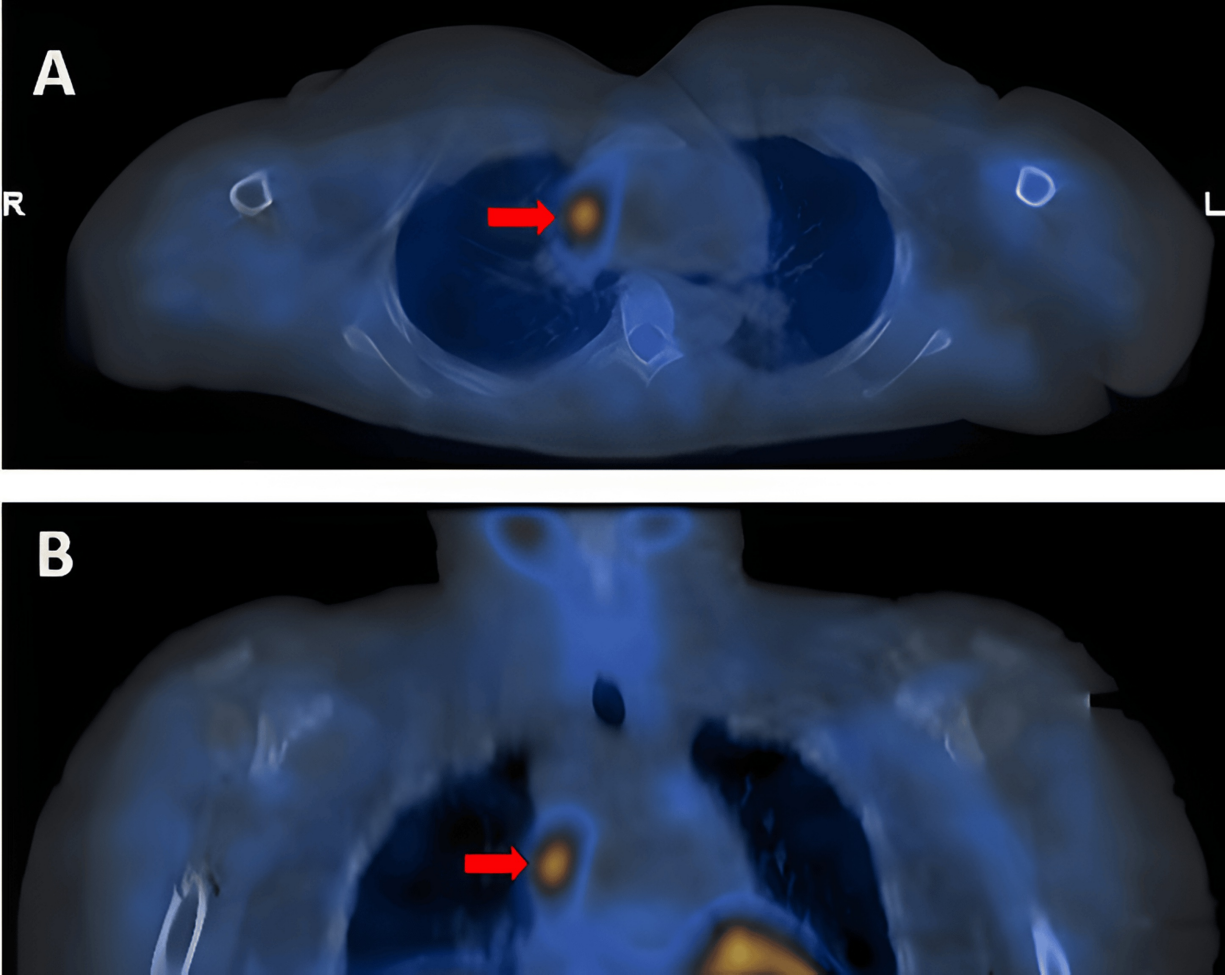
(A) Axial view of technetium-99m sestamibi single photon emission computed tomography/computed tomography. (B) Coronal view of technetium-99m sestamibi single photon emission computed tomography/computed tomography. The red arrow indicates the prominent increased activity in the medial aspect of the right mid to upper chest.
